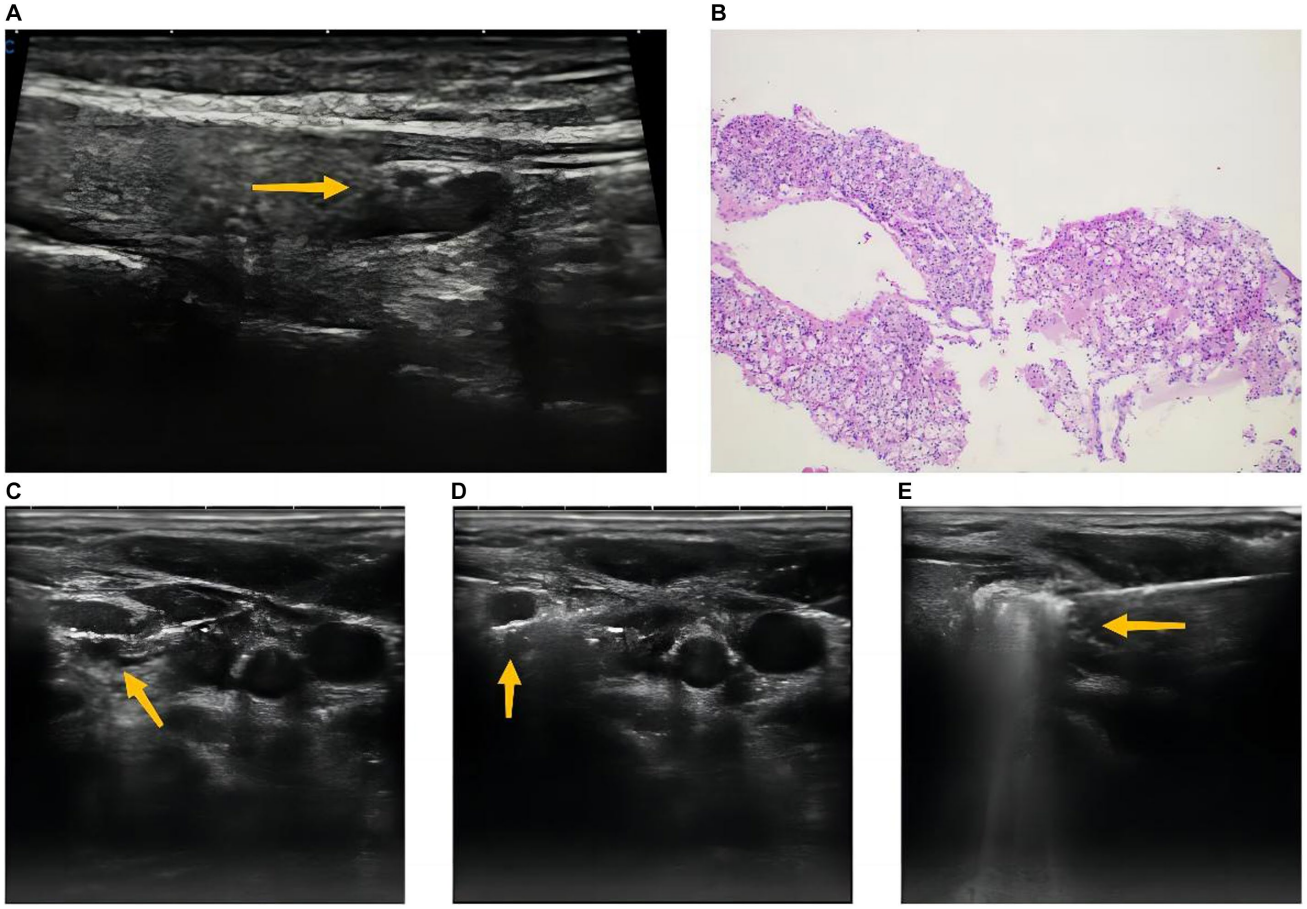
Ultrasound revealing a hypoechoic area with 1.1*0.3 cm on the dorsal side of the left inferior pole of thyroid (A). Parathyroid adenoma was confirmed by core needle biopsy, which was composed of chief, transitional, oncocytic, and water clear cells (B). Normal saline solution was injected into the region between the parathyroid adenoma and vital structures of the neck to protect recurrent laryngeal nerve, esophagus and trachea adjacent to the parathyroid adenoma (C). Antenna was located in the parathyroid adenoma (D). Ablation was finished until the entire nodule was covered with hyperechoic microbubbles (E).
